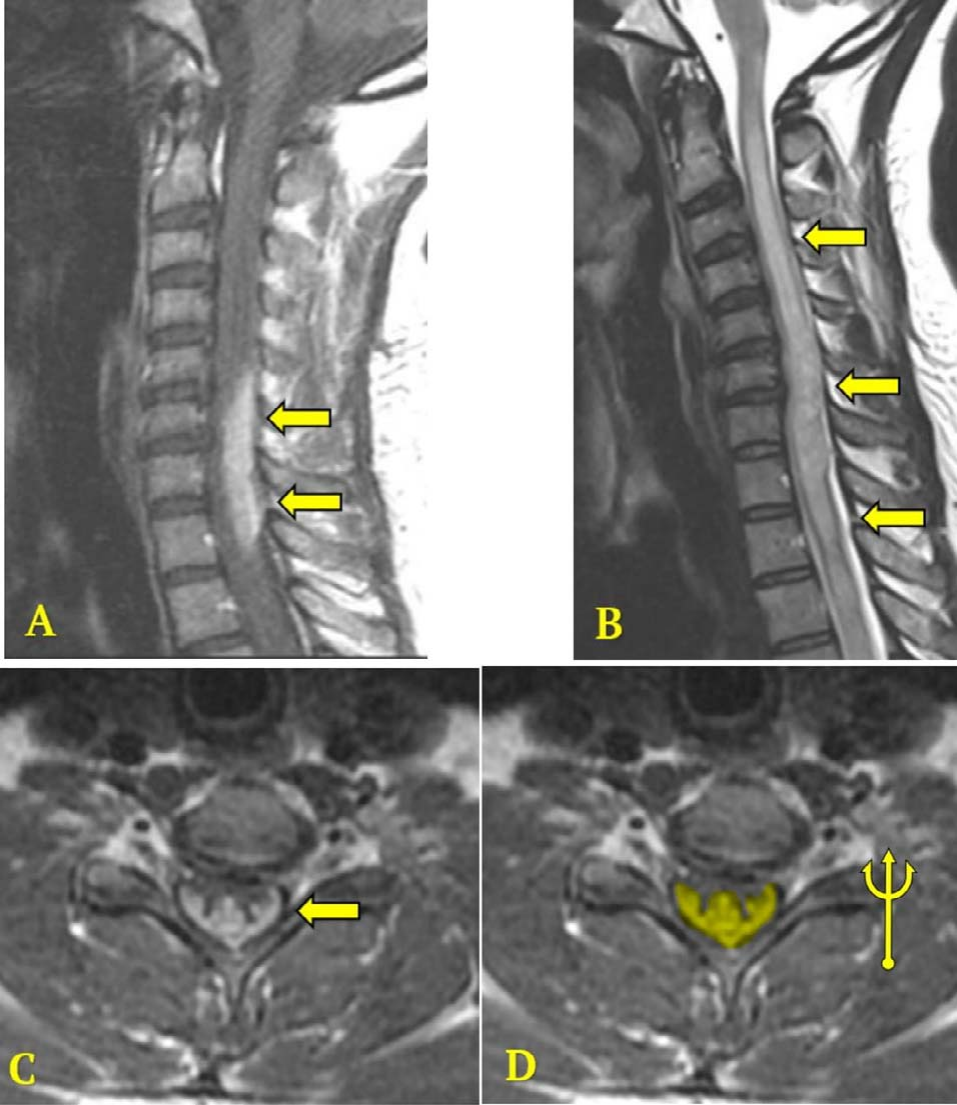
Magnetic resonance imaging of the spine. (A) Sagittal T1-weighted image with contrast shows extensive enhancement along the dorsal cord from C5 through T1 (arrows). (B) Sagittal T2-weighted image shows extensive signal abnormality throughout the visualized cervical and proximal thoracic cord (arrows). (C, D) Axial T1-weighted images with contrast show the characteristic trident sign (arrow and yellow highlight) corresponding to central cord enhancement with crescent-shaped dorsal subpial involvement.
